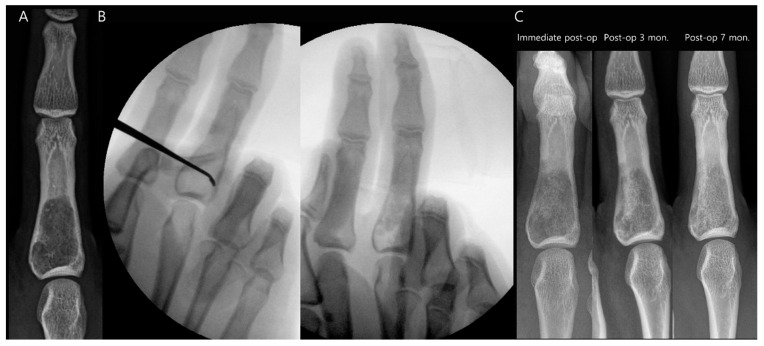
Intralesional curettage for enchondroma of the proximal phalanx of the middle finger. (A) Preoperative plain radiograph shows a well-defined osteolytic lesion containing chondroid calcification within the medullary cavity. (B) Intraoperative fluoroscopy shows complete curettage of the lesion, followed by autograft insertion. (C) Postoperative serial plain radiographs demonstrate successful incorporation of the graft 7 months after surgery.
